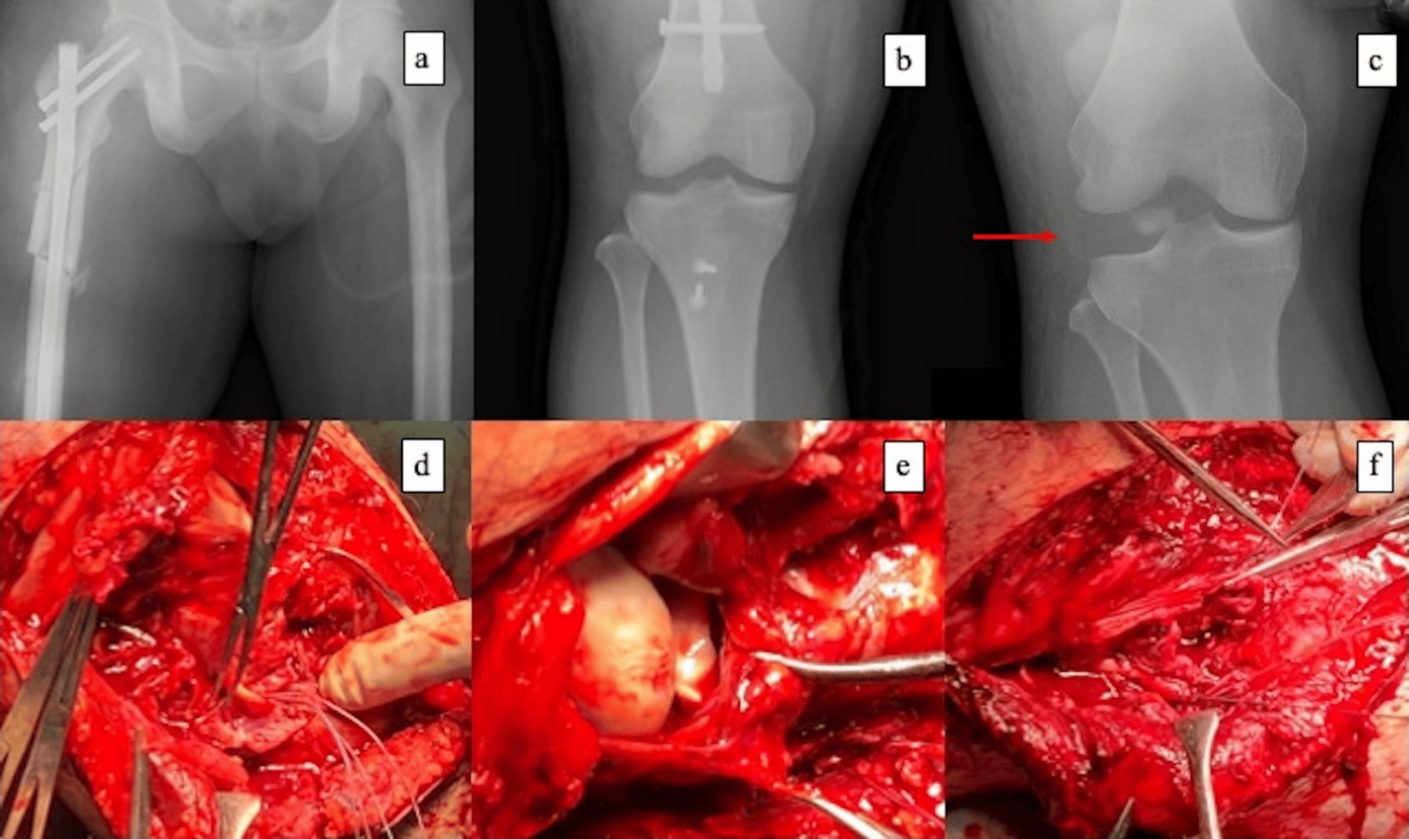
First-stage surgery: perioperative radiographs and intraoperative findings (a-b) Postoperative radiographs showing femoral shaft fixation with an intramedullary nail and tibial tuberosity fixation with cannulated cancellous screws. (c) Preoperative varus stress radiograph demonstrating grade III lateral opening, consistent with severe lateral-sided instability. (d-f) Intraoperative findings and repair; The lateral collateral ligament was torn near its fibular insertion and was repaired using a suture anchor placed in the fibular head. A longitudinal tear of the lateral meniscus (midbody) was repaired under direct visualization. The lateral capsule was then repaired.
